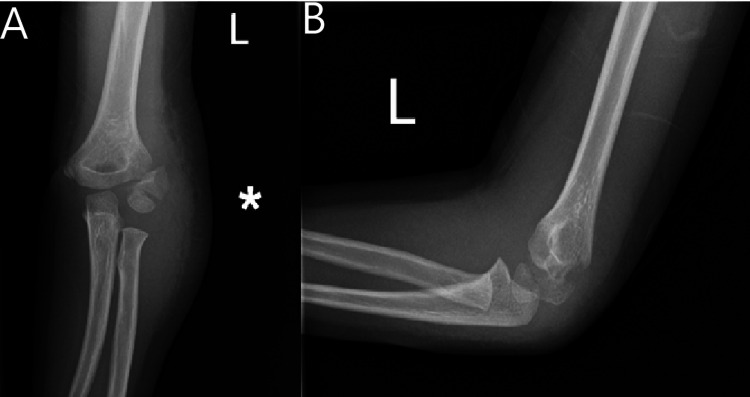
(A) Anteroposterior and (B) lateral radiographs of the left elbow showing a displaced medial condyle fracture (Case 1) The * in the figure is the local radiography department's way of indicating there is a deformity with the radiograph, in this case, to indicate the Milch type 2 fracture. It was already present on the radiograph and not added on subsequently.
